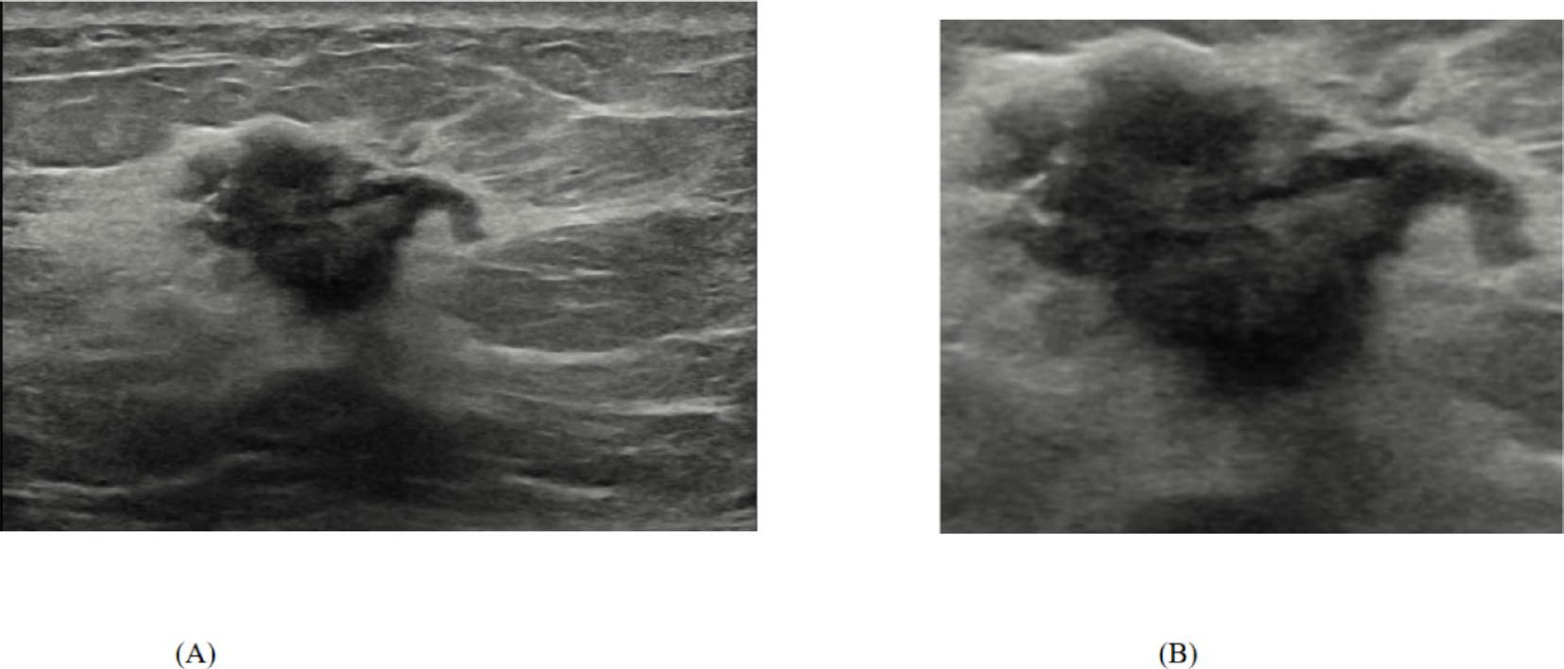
A shows the original breast ultrasound image. B displays the corresponding bounding box annotation used to localize the lesion region for subsequent analysis.
